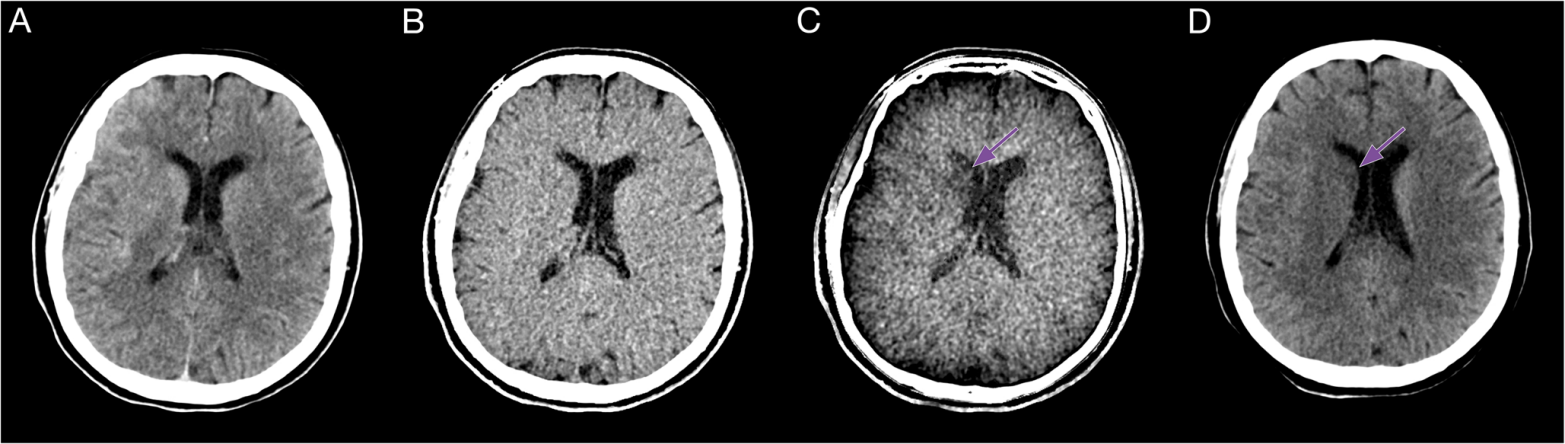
Example of a caudate nucleus infarction in the BW (A), VNC (B) and EM (C) series. On the EM, the infarction displays pronounced contrast relative to nearby brain parenchyma (arrow). Follow-up CT with infarction delineation (D, arrow)
